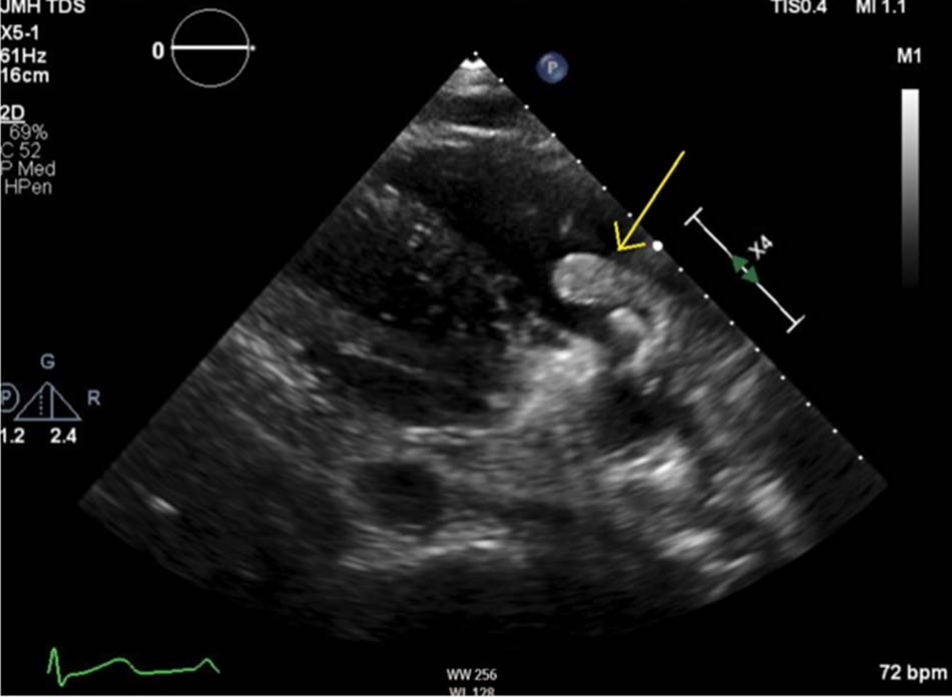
Parasternal right ventricular inflow view showing mobile thrombus (arrow).
